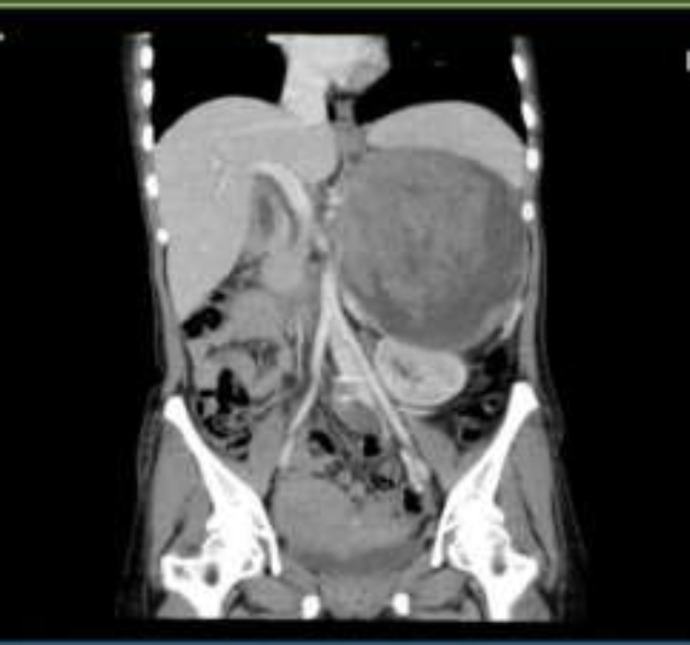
Abdominal and pelvic CT scan showing a heterogeneous mass with in the left adrenal anatomical location in the vicinity of the tail of the pancreas
